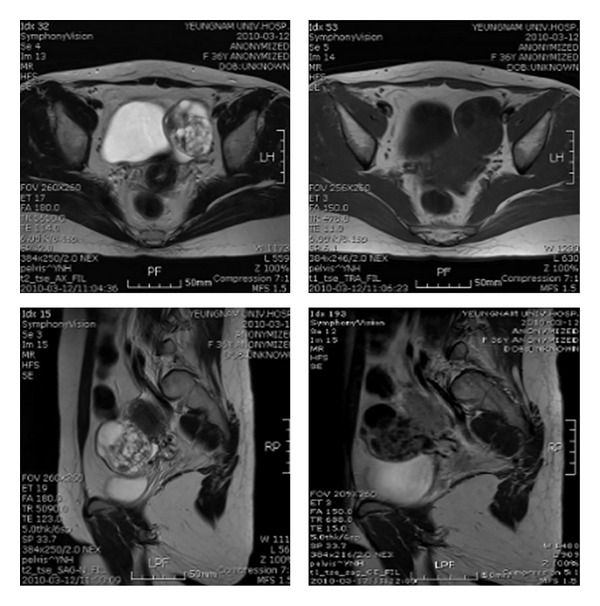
Pelvic MRI before surgery. Image showed approximately 6 cm diameter mass with numerous small cysts and fibrous septal structure in the left ovary.
